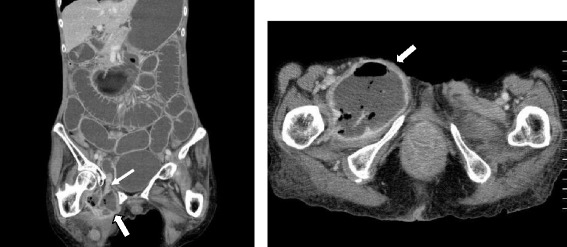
Computed tomography (CT) revealed a right obturator hernia (*thin arrow*) and abscess spreading to the right thigh (*bold arrow*)
